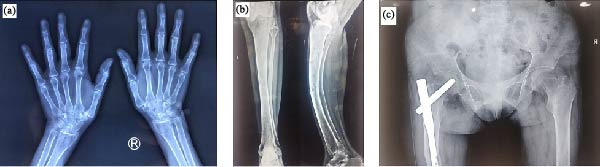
(a) Bilateral hand radiographs demonstrating subperiosteal resorption along the middle phalanges. (b) X‐ray of the left tibia (AP and lateral views) showing a well‐defined lytic lesion in the mid‐shaft with a narrow zone of transition and surrounding sclerosis, suggestive of a healed Brown’s tumour. (c) X‐ray pelvis showing a left femoral implant in situ with pubic symphysis resorption and generalised osteopenia characterised by coarse, prominent trabeculae, most marked in the bilateral iliac bones.
